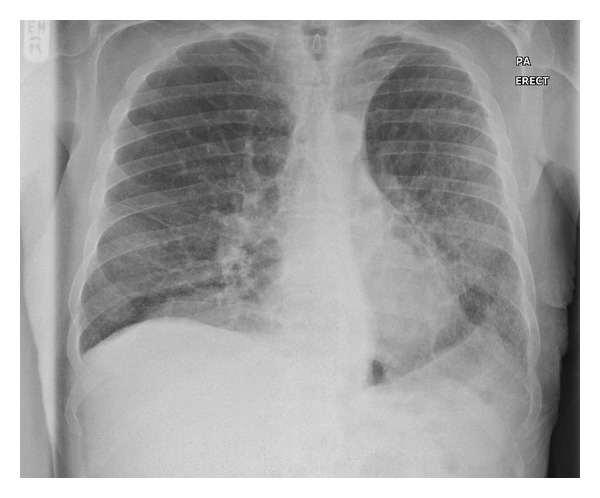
Chest X-ray on day one of admission.
